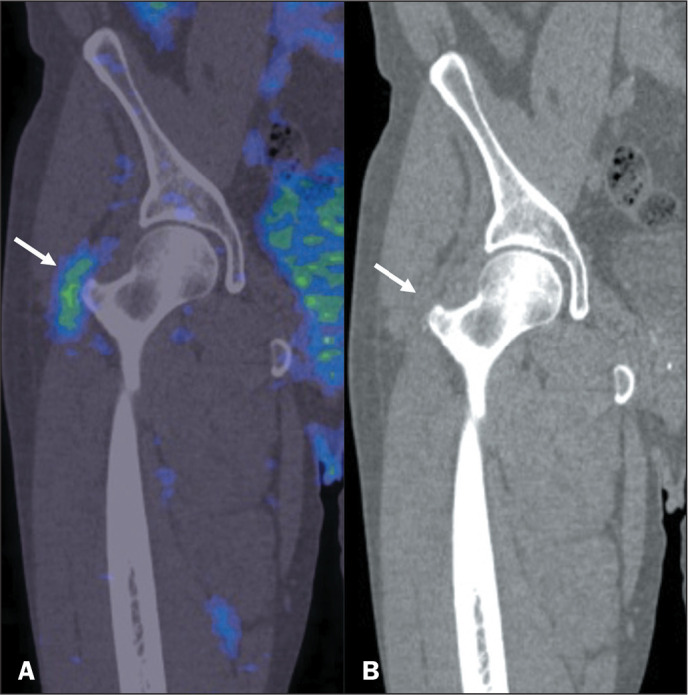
^68^Ga-PSMA PET/CT of a 60-year-old male patient, performed
